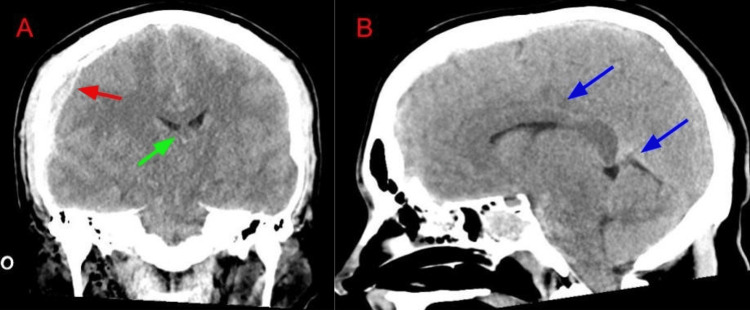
Noncontrast CT head coronal (A) and sagittal (B). Comminuted fracture of the right temporal bone, extending to the right occipital bone. Large subdural hematoma along the right cerebral convexity (red arrow), causing midline shift to the left by approximately 8 mm (green arrow). Diffuse subarachnoid hemorrhage (blue arrows).
